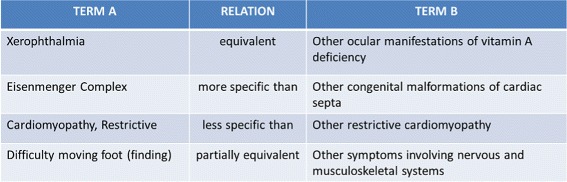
Examples of ambiguous correspondences between SNOMED-CT and ICD9CM
